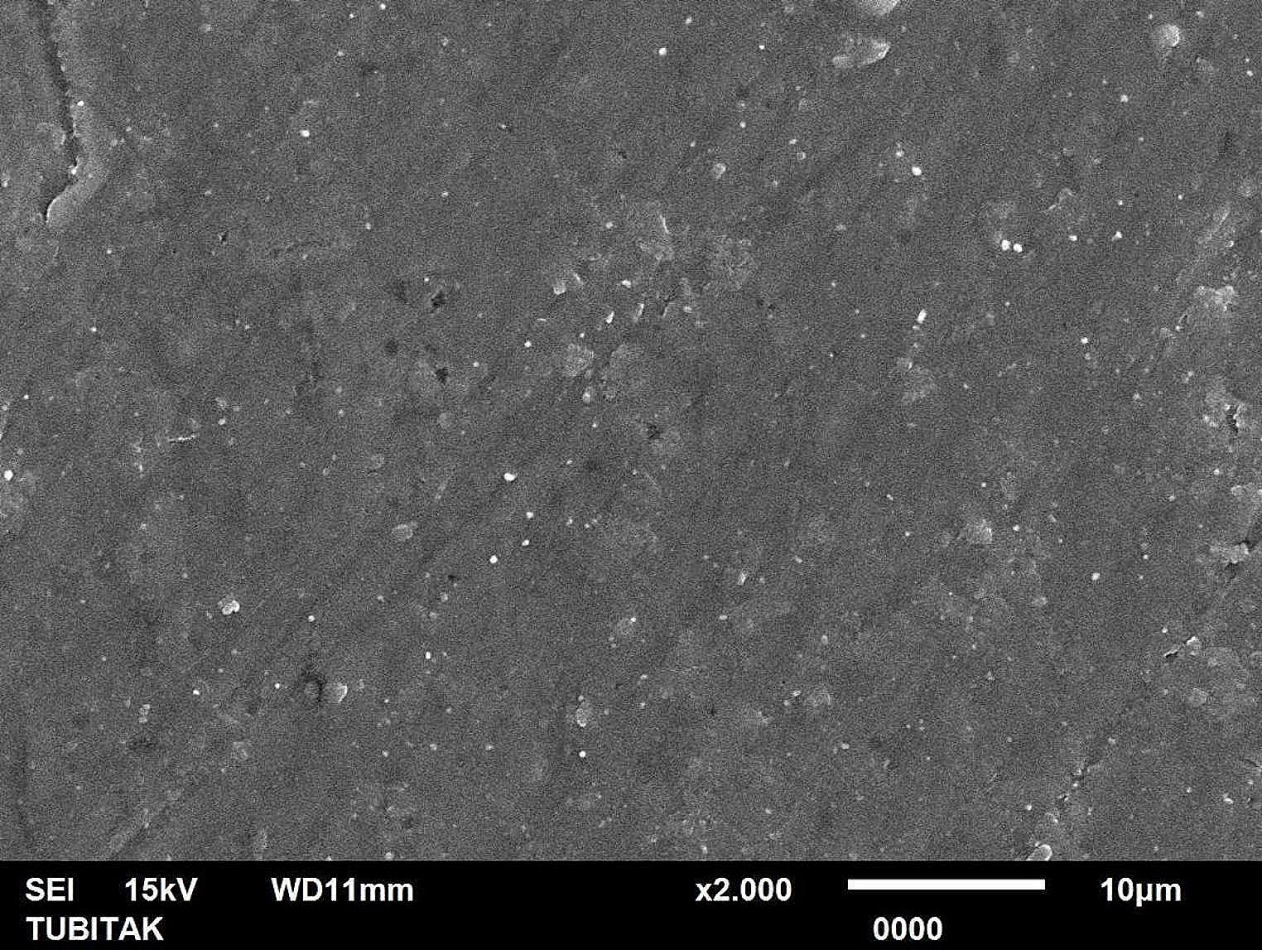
SEM image of the new generation ultrasonic tip-applied treatment group (x2000 magnification)
